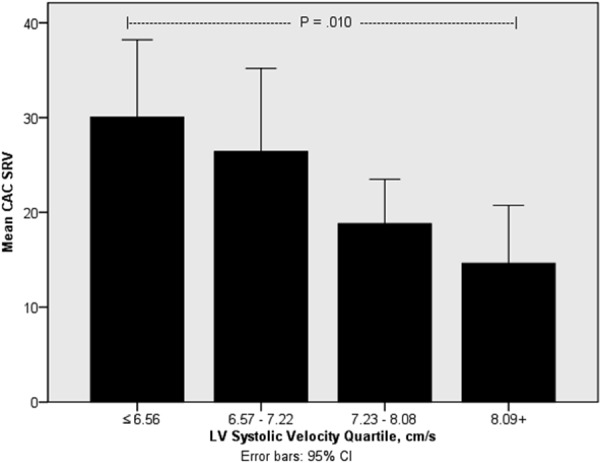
Mean coronary artery calcification (CAC) square root of the volume by left ventricular (LV) systolic velocity quartile. CAC scores were normalized as the square root of the volume and expressed according to peak LV systolic velocity quartiles expressed as cm/s using tissue Doppler imaging. CAC severity is associated with a progressive decrease in the peak LV systolic velocity obtained by tissue Doppler imaging reaching statistical significance between the lowest and highest quartile groups. These findings suggest the development of subclinical LV systolic dysfunction with CAC severity.
